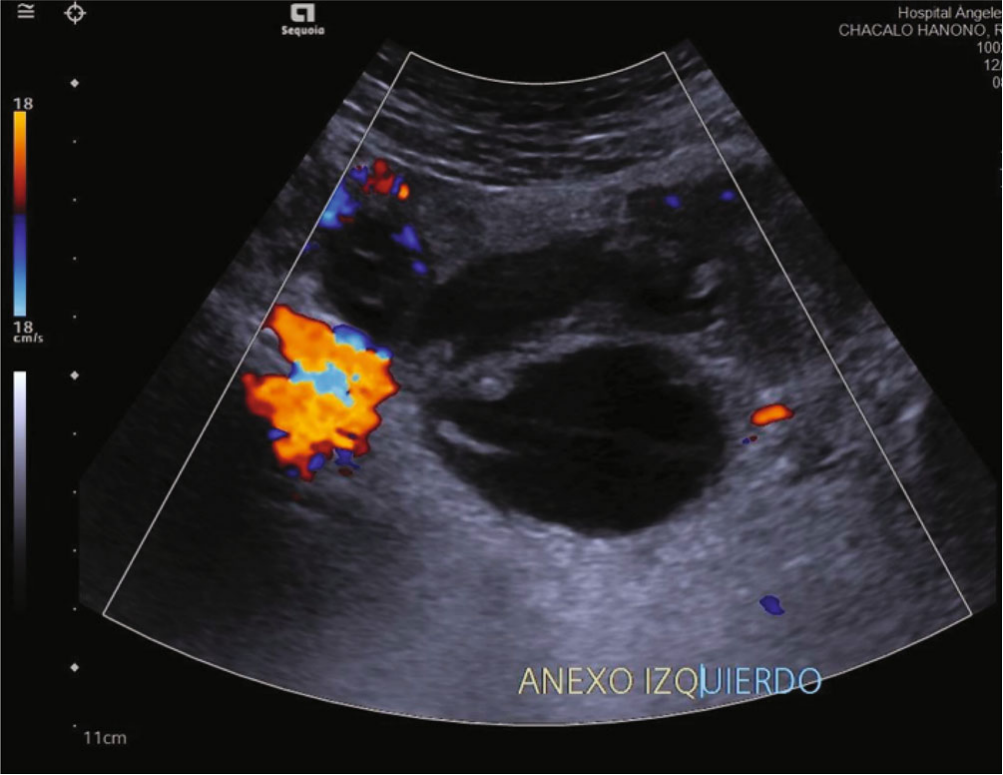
Collection in the pelvic cavity of heterogeneous content, without vascularity on color Doppler of 5.9 cm × 5.6 cm × 4.9 cm with an approximate volume of 86 cc.
